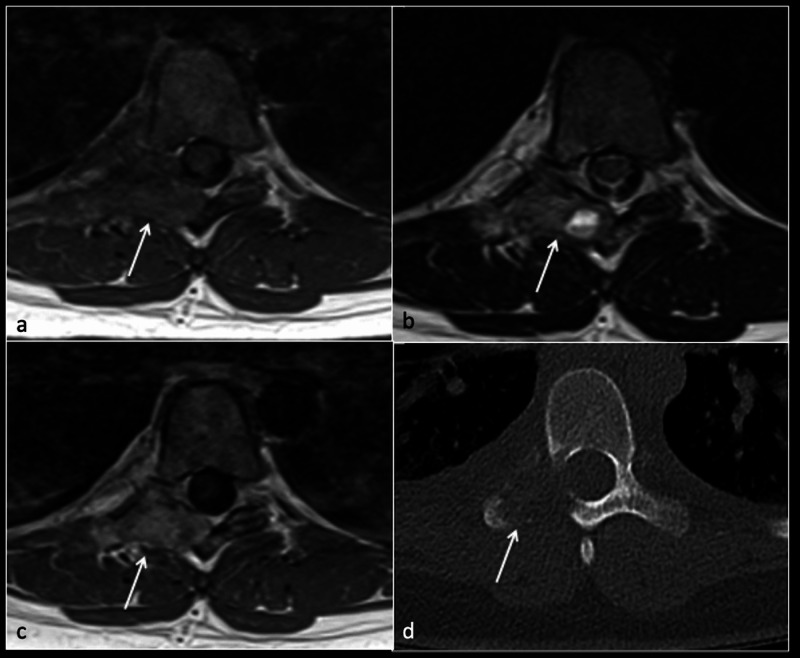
Axial T1 (a), T2 (b), T1 post-contrast (c), and CT (d) showing tumour (arrow) in the right pedicle and lamina with fluid level CT: computed tomography
